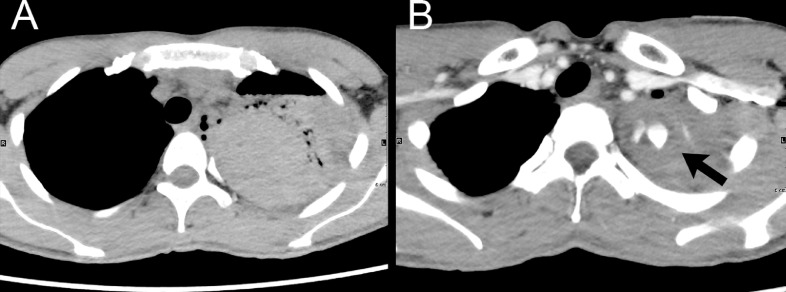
Computed tomography (CT) before transcatheter arterial embolization. (A) Left massive hemothorax was observed on CT. (B) Contrast leakage into the left thoracic cavity indicated active bleeding (arrow).
